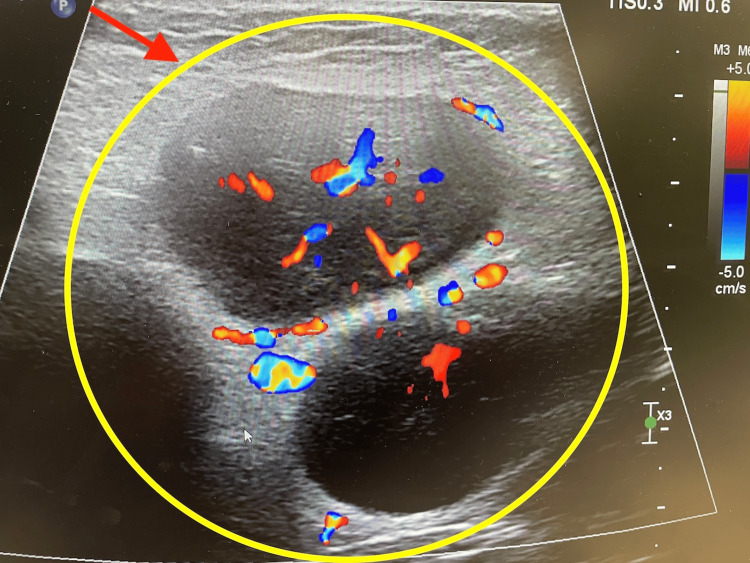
Ultrasound with color Doppler imaging demonstrating increased vascular flow
